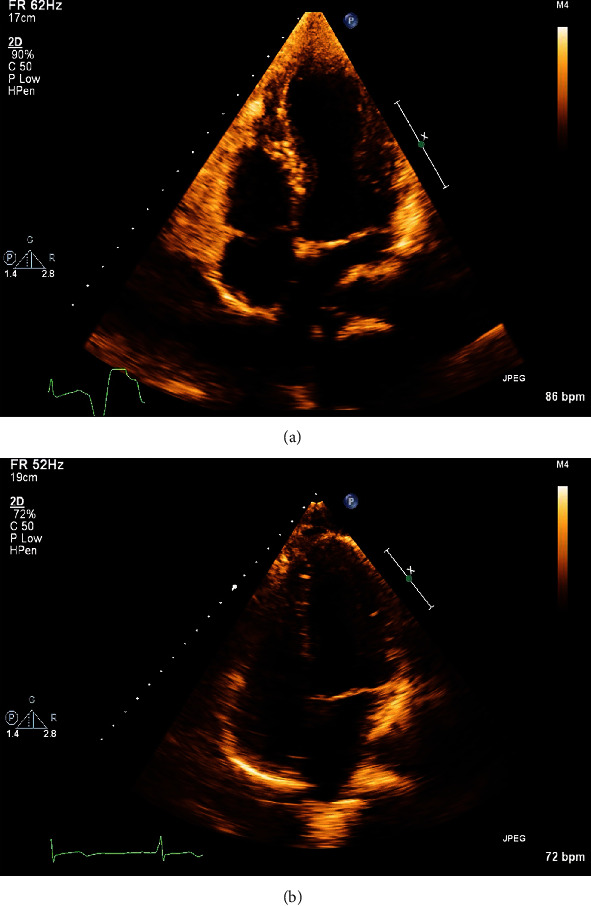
(a) TTE apical 4-chamber view in systole, demonstrating apical ballooning, suggestive of Takotsubo's stress-induced cardiomyopathy. (b) 14 days later: TTE apical 4-chamber view in systole, demonstrating resolution of patient's Takotsubo's stress-induced cardiomyopathy.
